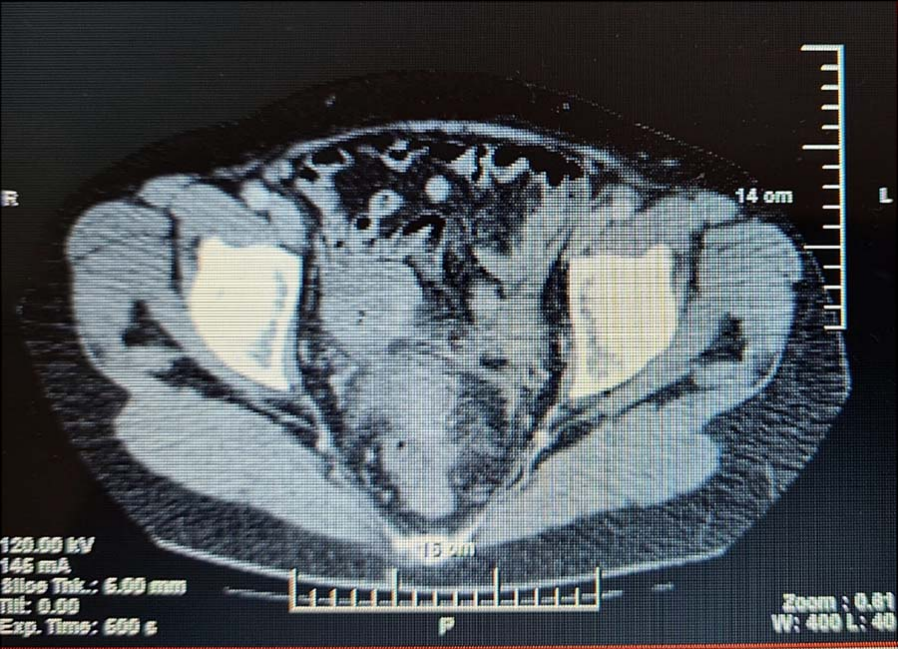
CT of the abdomen shows stenosing of the sigmoid colon.
